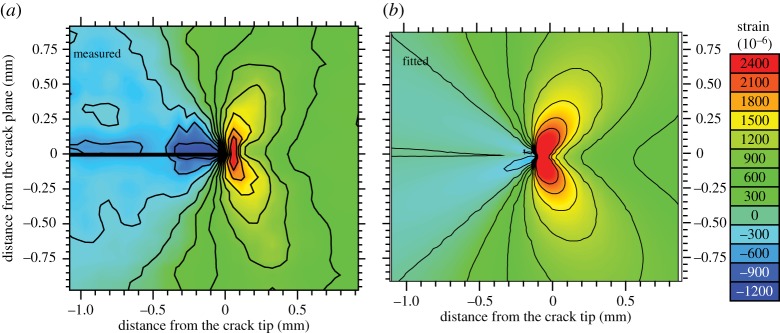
The measured (*a*) and best-fit (*b*) elastic strain fields (in 10^−6^) for the crack-opening (*ε*_*yy*_) direction at *K*_Imax_=6.6 MPa√m for an Al 5091 CT sample. The LEFM best-fit crack-tip strains correspond to a crack-tip stress intensity of *K*^eff^_Imax_=6.11 MPa√m,K^eff^_*IImax*_=0.33MPa√m (table 1) [22]. (Online version in colour.)
